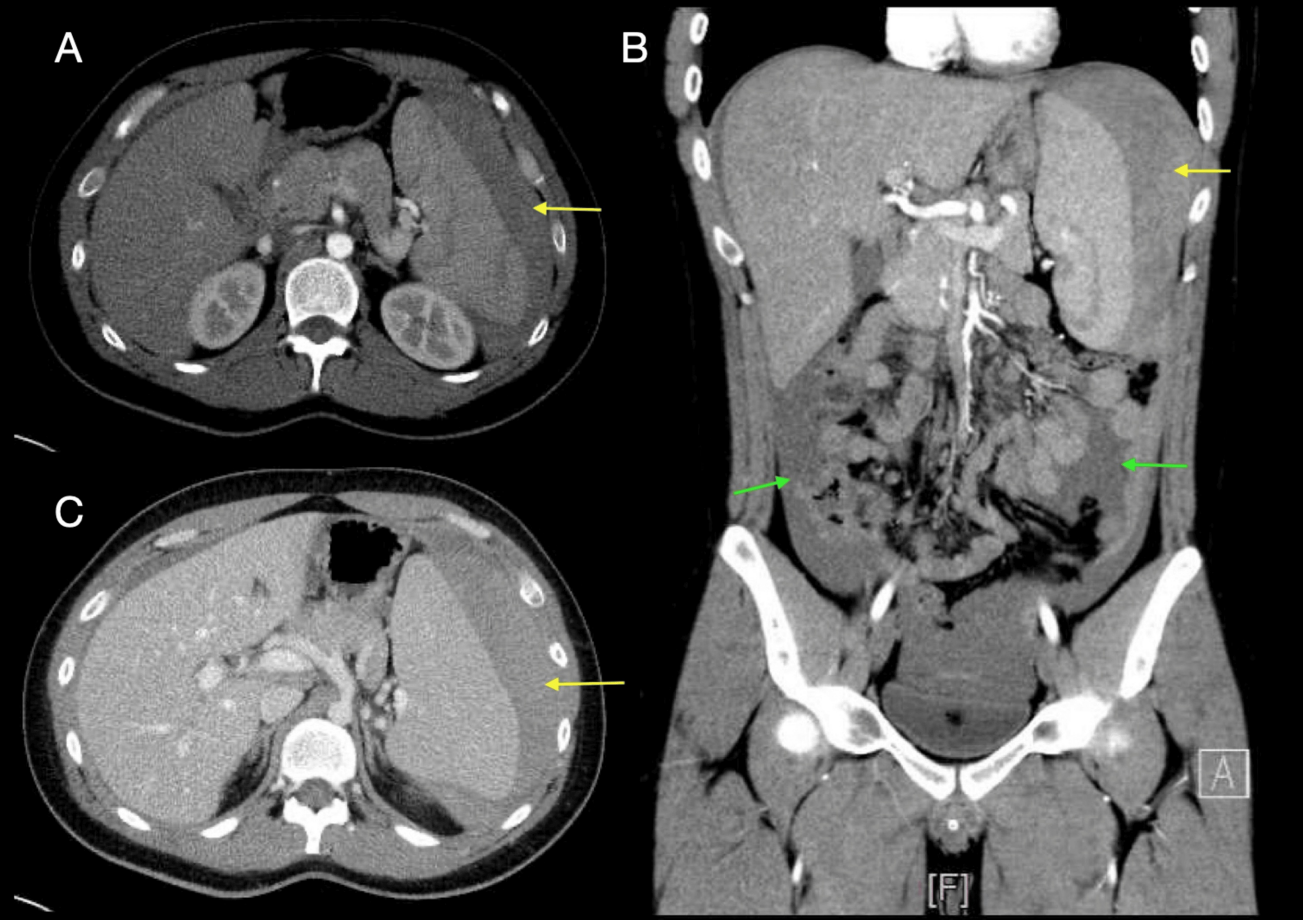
Computed tomography of the patient showing the splenomegaly and splenic rupture, perisplenic hematoma, and hemoperitoneum. (A) Axial arterial-phase image, (B) sagittal arterial-phase reconstruction, (C) axial venous-phase image. The yellow arrows indicate a perisplenic hematoma, and the green arrows indicate free intraperitoneal fluid, hemoperitoneum.
